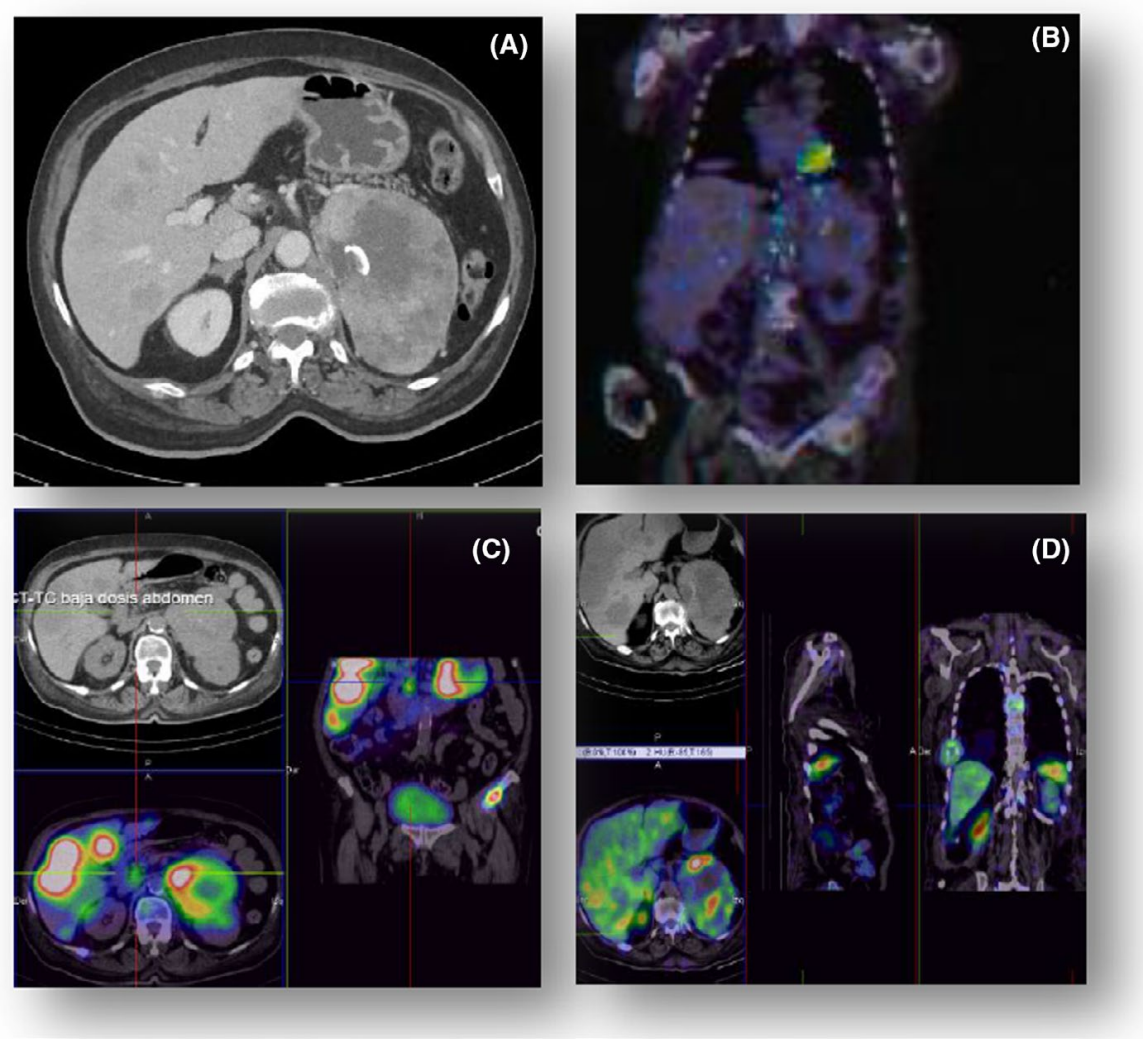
Contrast‐enhanced abdominal computed tomography (1A), F‐18 FDG PET/CT (1B), 123I‐MIBG scintigraphy (1C), and Technetium‐99m Octreotide scintigraphy (1D) of malignant pheochromocytoma
